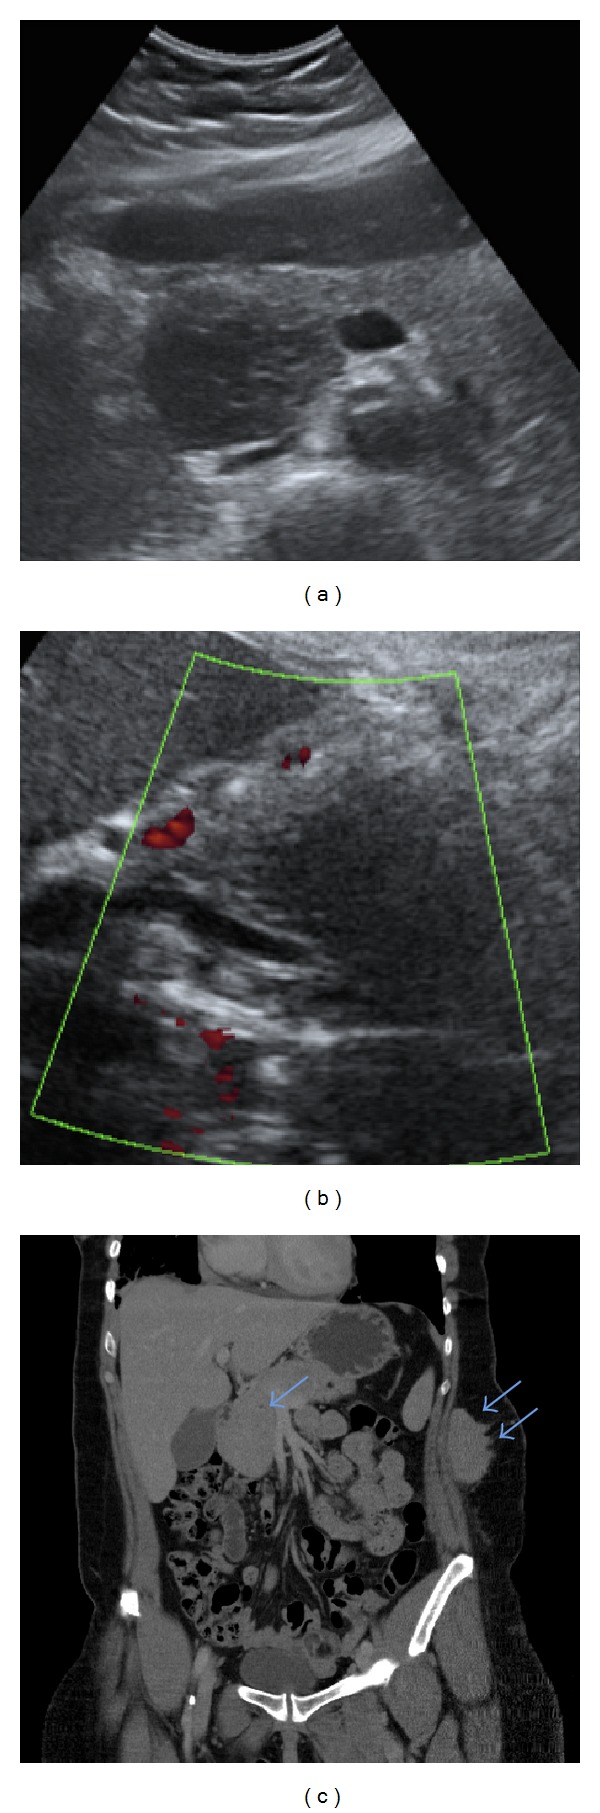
Abdominal ultrasonography and tomography*: abdominal ultrasonography showed a hypoechoic, ill-defined, pancreatic mass measuring 49 × 36 mm (a). Although the mass affected the pancreatic head, the patient manifested no jaundice. This is justified by the absence of bile duct compression (b). Coronal abdominal CT imaging revealed a solid ill-defined mass in the pancreatic head (single arrow) (c) and another similar lesion in the subcutaneous tissue of the left lateral abdominal wall (double arrows) (c).
